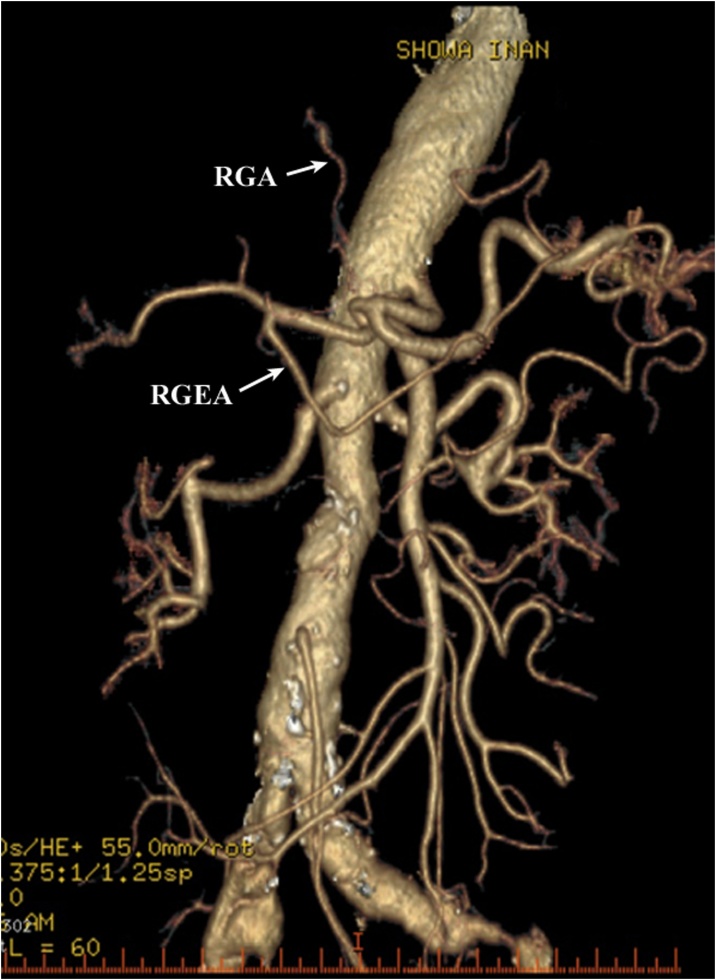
Preoperative 3-dimensional angiography reconstructed from the computed tomography scan; the right gastroepiploic and right gastric arteries are shown supplying blood to the gastric tube.
